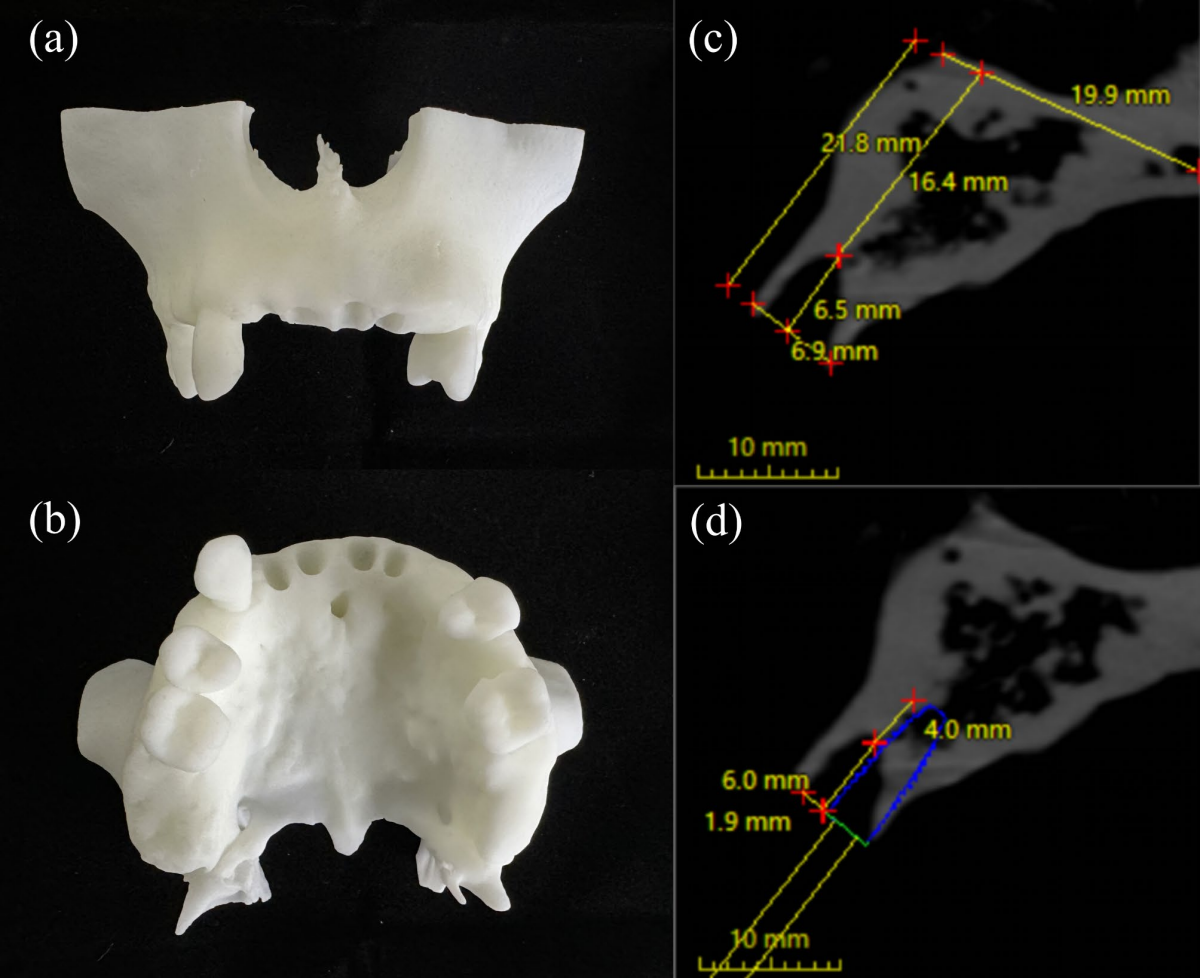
Model and digital plan (a) Occlusal view of the resin model; (b) Buccal view of the resin model; (c) Measurements of the extraction socket on sagittal plane of CBCT scans in the anterior maxilla; (d) Measurements of the planned implant relationship to the extraction socket on sagittal plane of CBCT scans in the anterior maxilla. CBCT, cone-beam computed tomography
